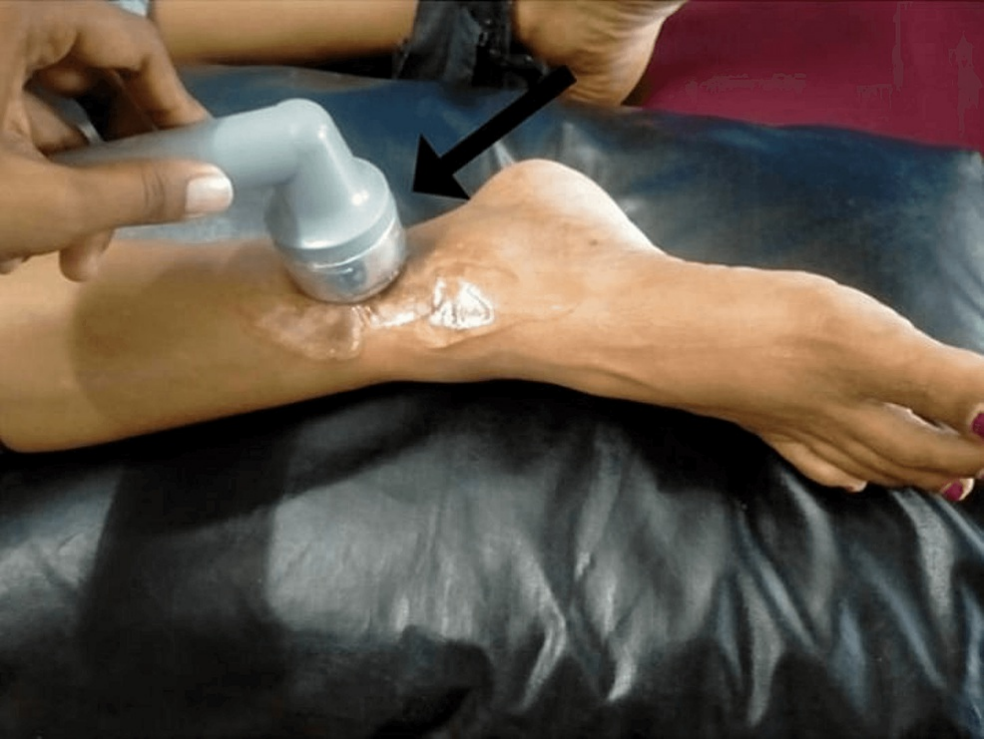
Patient undergoing ultrasound therapy for a scar mobilization
